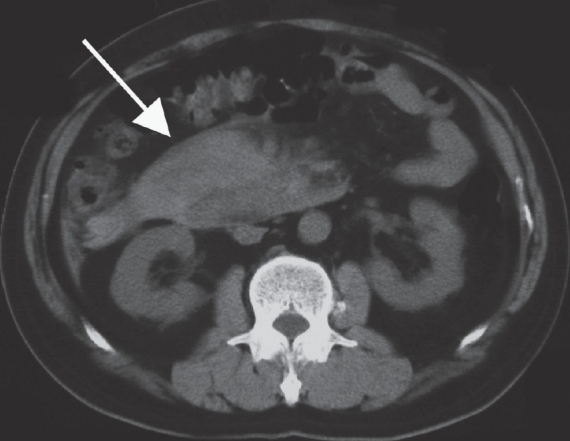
Plain axial CT scan shows a massive retroperitoneal hematoma (arrow) around the pancreatic head
